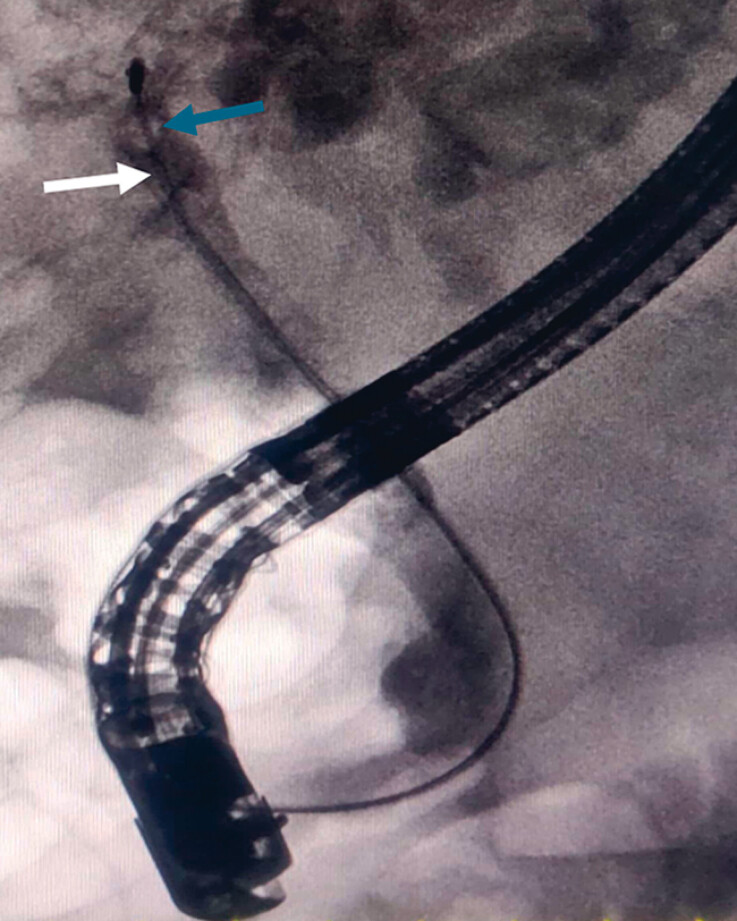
X-ray imaging revealed the impaction of the basket (indicated by the white arrow), along with the cross-section of a drainage catheter (indicated by the blue arrow).
